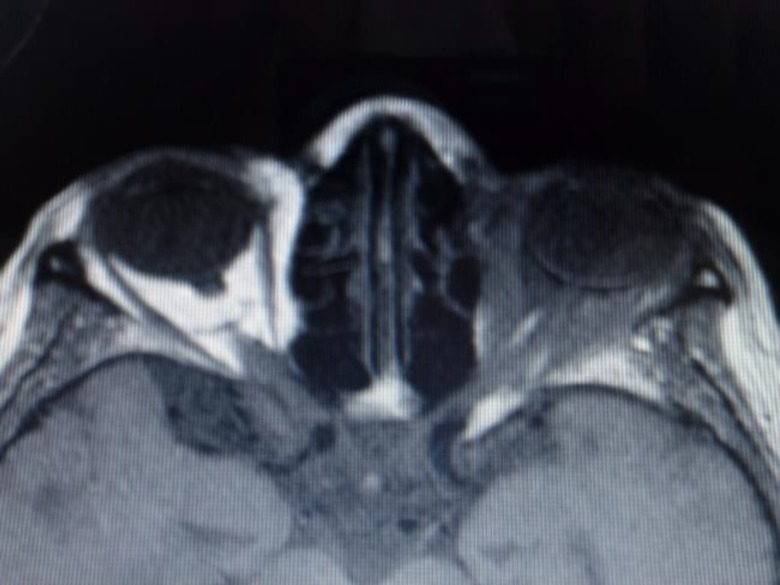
Magnetic Resonance Imaging of the Orbit Demonstrated Infiltration of Orbital Fat and the Extra-Ocular Muscles
